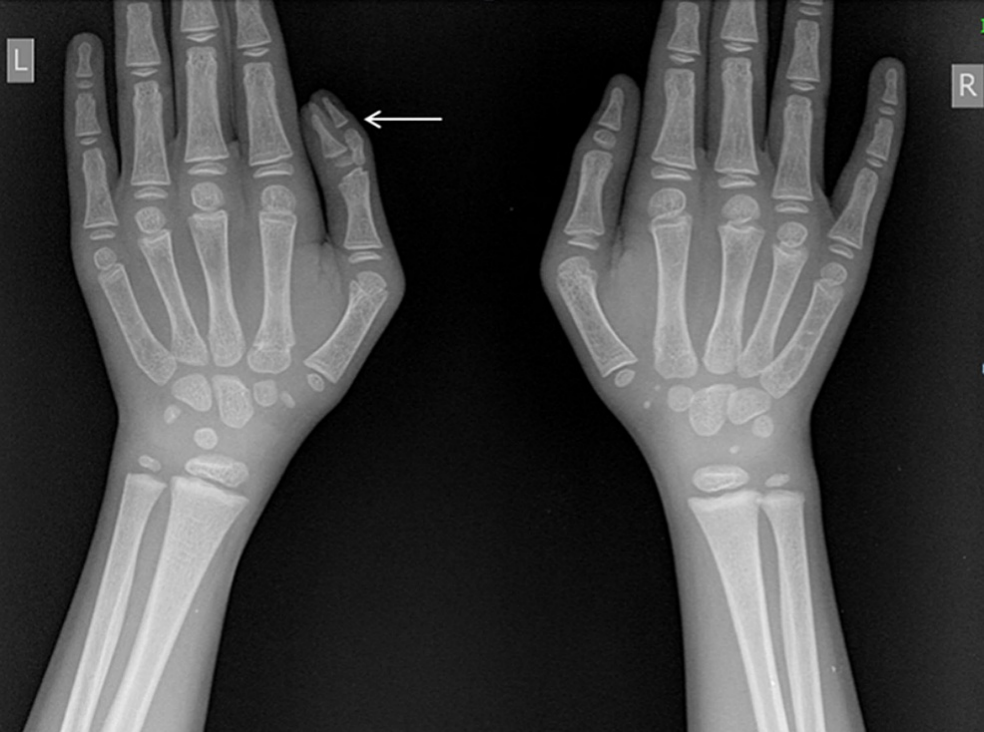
Radiograph of bilateral hands demonstrating polydactyly in the left thumb.
